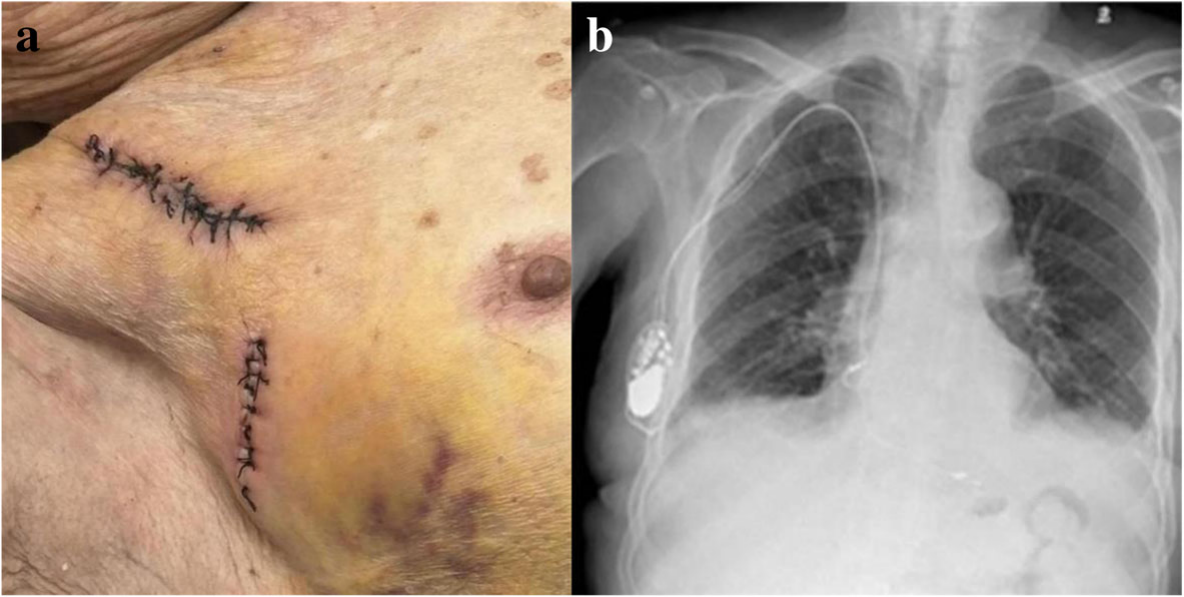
a Appearance of the wound after reimplantation. b Postoperative chest X-ray showed the generator was reimplanted in different position on the same side
